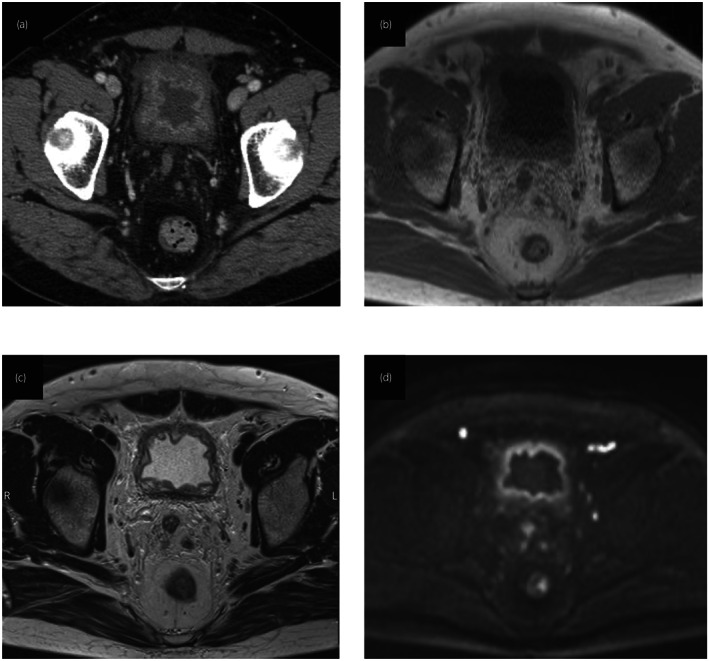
Enhanced computed tomography shows an irregular and thick enhanced bladder wall (a). The thickened bladder wall displayed homogeneous hyperintensity relative to the muscle on T1WI (b) and hypointensity on T2WI (c), and diffusion was slightly restricted on DWI (d).
